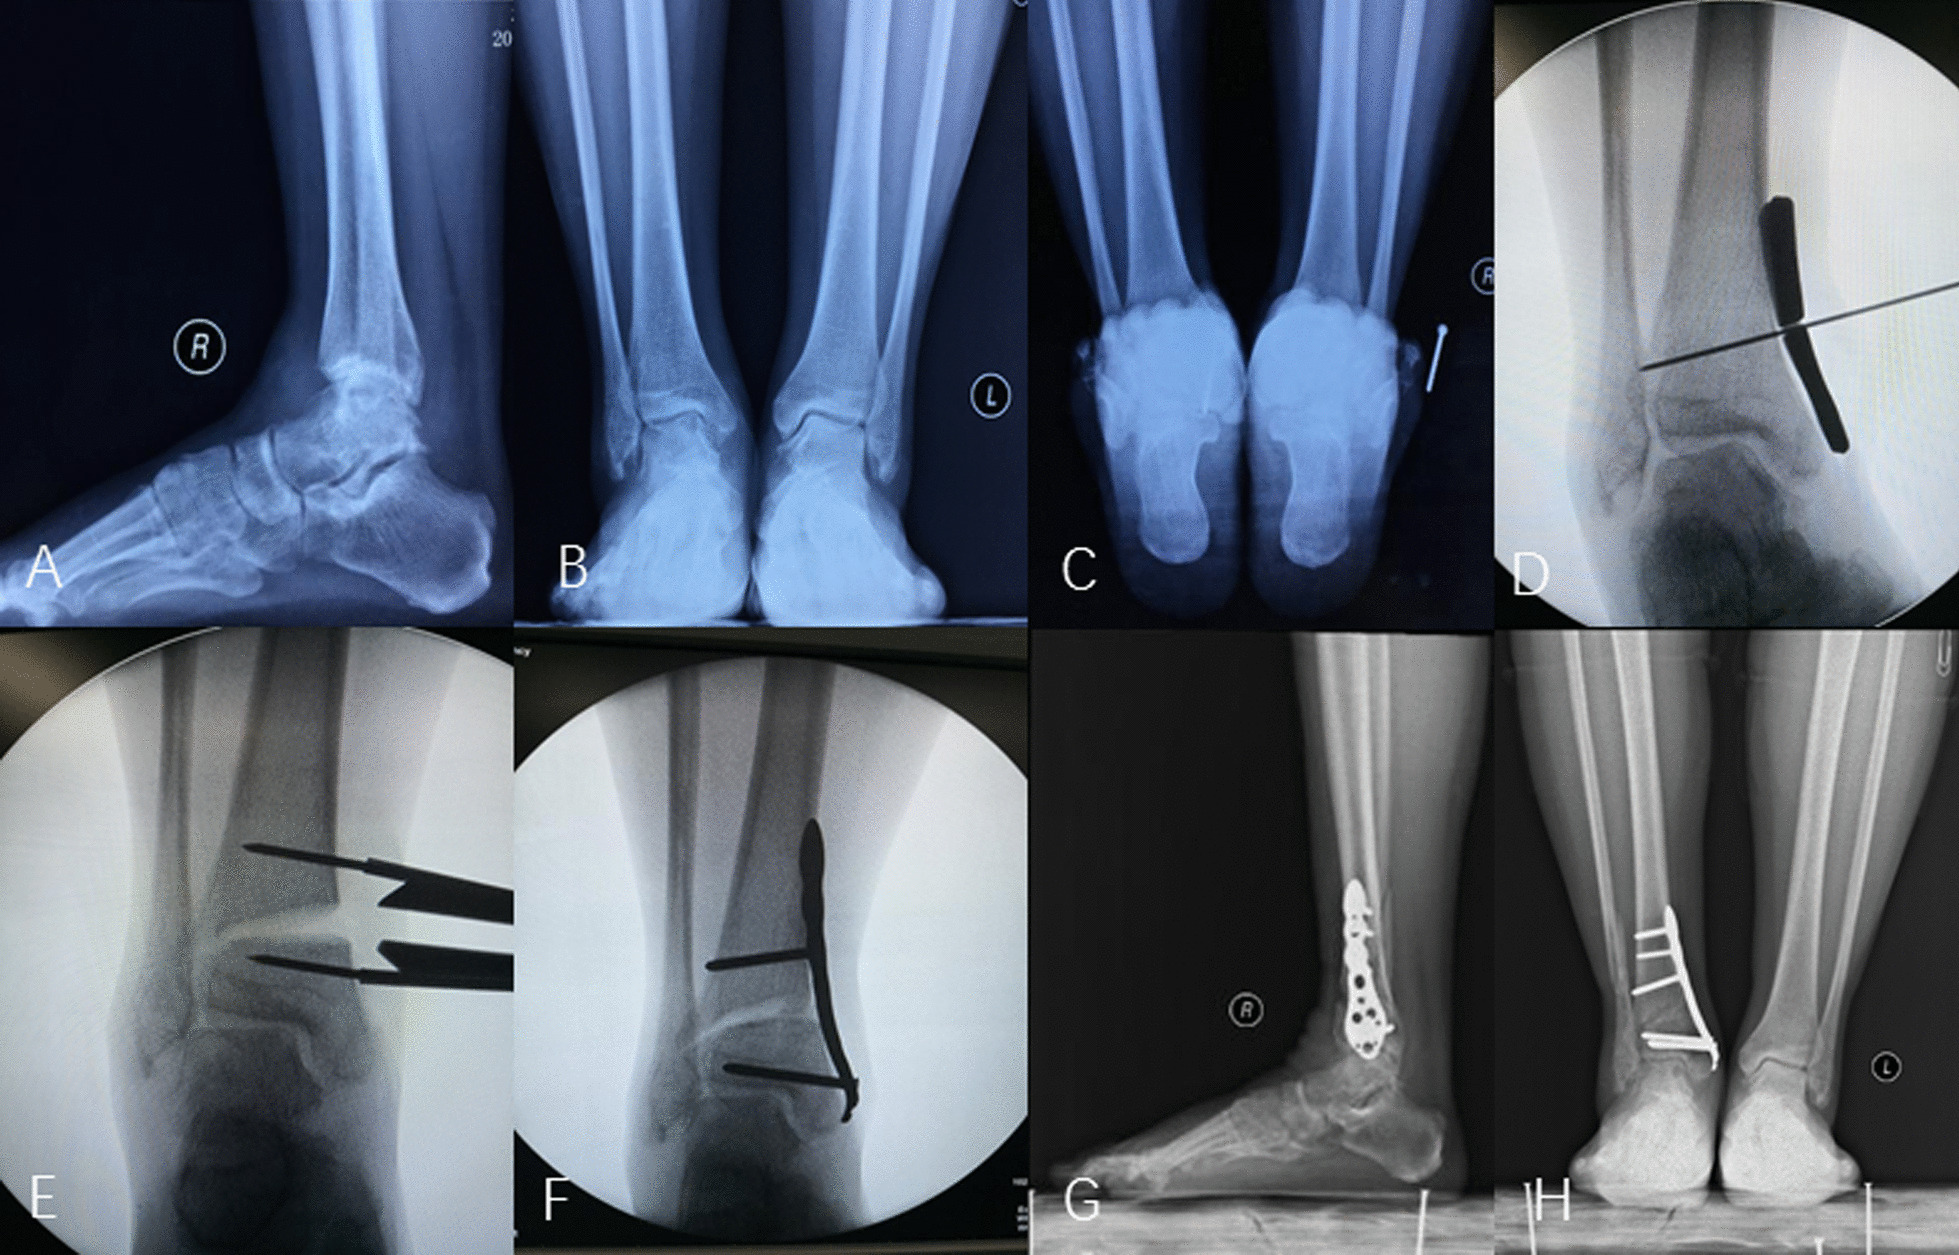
Depicts a female, 48 years, who had right Takakura-Tanaka stage 3 varus ankle osteoarthritis and underwent supramalleolar osteotomy to reconstruct the mechanical axis. Preoperative radiographs A–C (lateral, anteroposterior, and calcaneal axial view) showed the ankle varus deformity and osteoarthritis; D–F showed the operative procedure process (localization of supramalleolar osteotomy, widening the gap, and internal fixation). G, H Showed the improved force line of the ankle joint and the articular space
